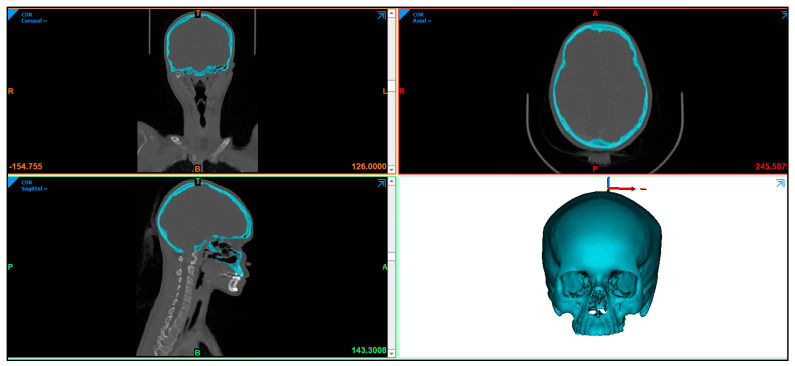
Radiographic image displaying the skull anatomy and the creation of 3D image model.
